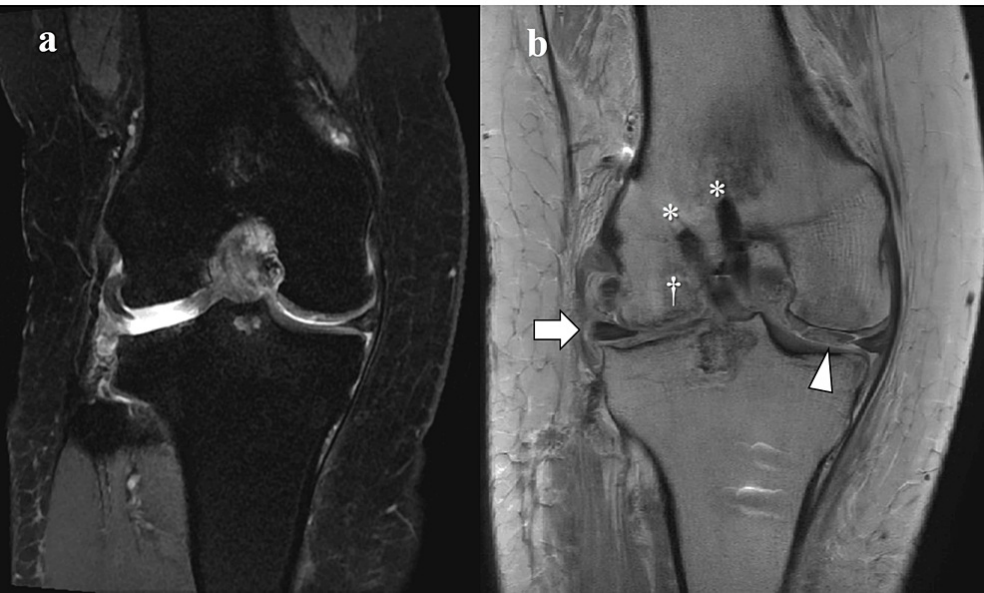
Coronal views of MRI (Case #4) - image 1 a. Preoperative proton density-weighted image (TR2427, TE 36.63, FOV:160). b. T2-weighted image (TR6000, TE 8.75, FOV:150) 1 month postoperatively *: Reconstructed anterior cruciate ligaments. †: Osteochondral autograft. White arrow: transplanted meniscal allograft. Arrowheads: autologous chondrocyte transplantation MRI: magnetic resonance imaging
